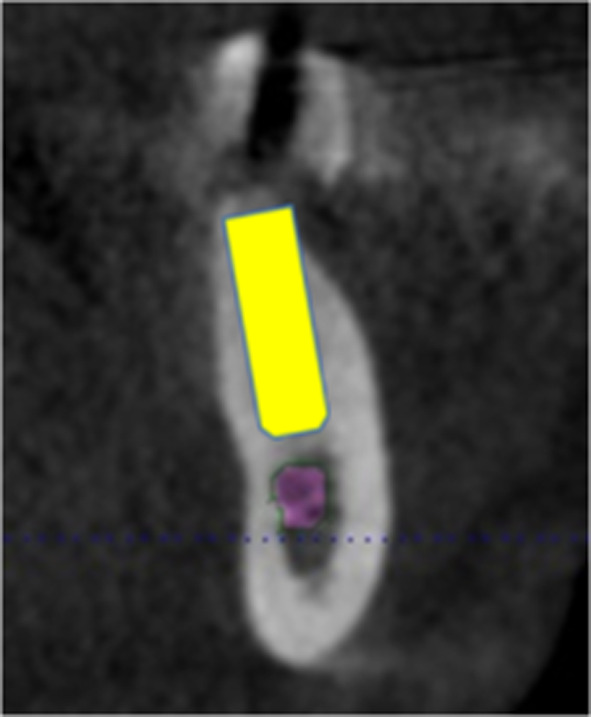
The same patient’s (Fig. 1) CBCT with the virtually planned implant in region 37 showed inconspicuous jaw contour
